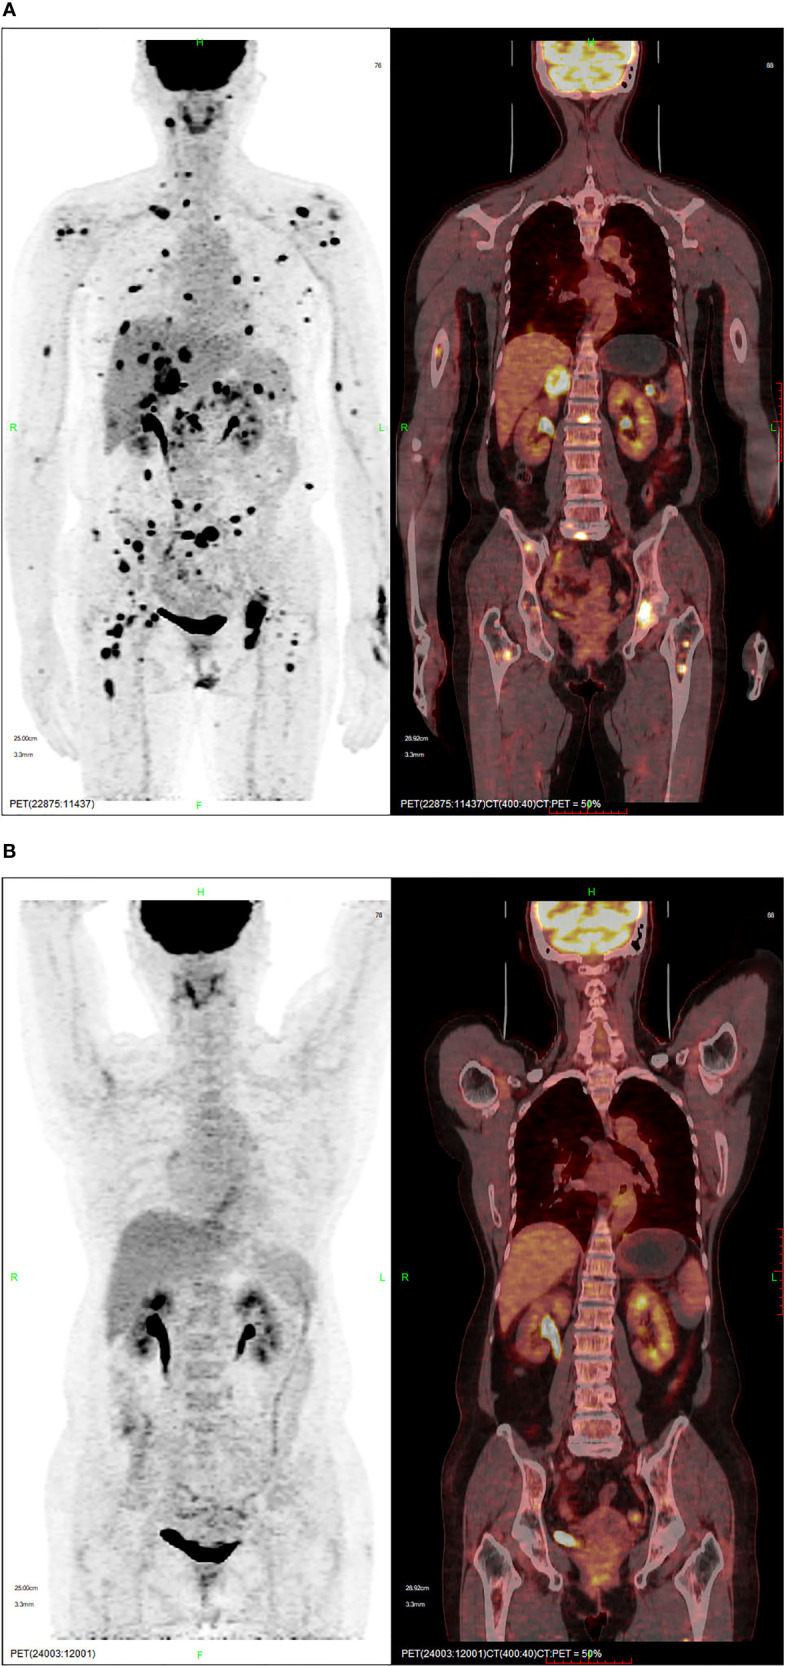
PET-CT showing (A) the lesion and high FDG uptake in November 2020, and (B) complete remission following crizotinib in August 2021.
